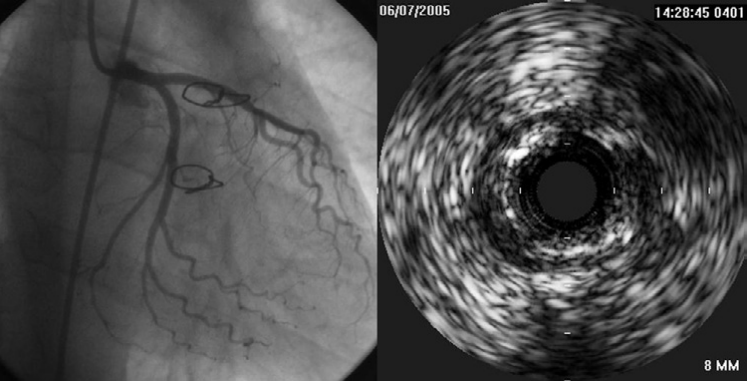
Graft coronary artery imaging- appearance by angiography and intravascular ultrasound 1 month after follow-up. Excellent angiographic result demonstrating concordance with the pre-transplant potential donor stress echo (no inducible ischemia). The left panel shows angiogram obtained on the transplanted heart 1 month after engraftment. Angiographic imaging of the midportion of the LAD only shows minor lumen irregularities within the vessel. The right panel shows intravascular ultrasound images obtained in the same coronary segment. (images from Prof Claudio Rapezzi and Dr Carlo Magelli, responsible of the Clinical Heart Transplant Program, University Cardiology Division, S. Orsola Hospital, Bologna, Italy)
